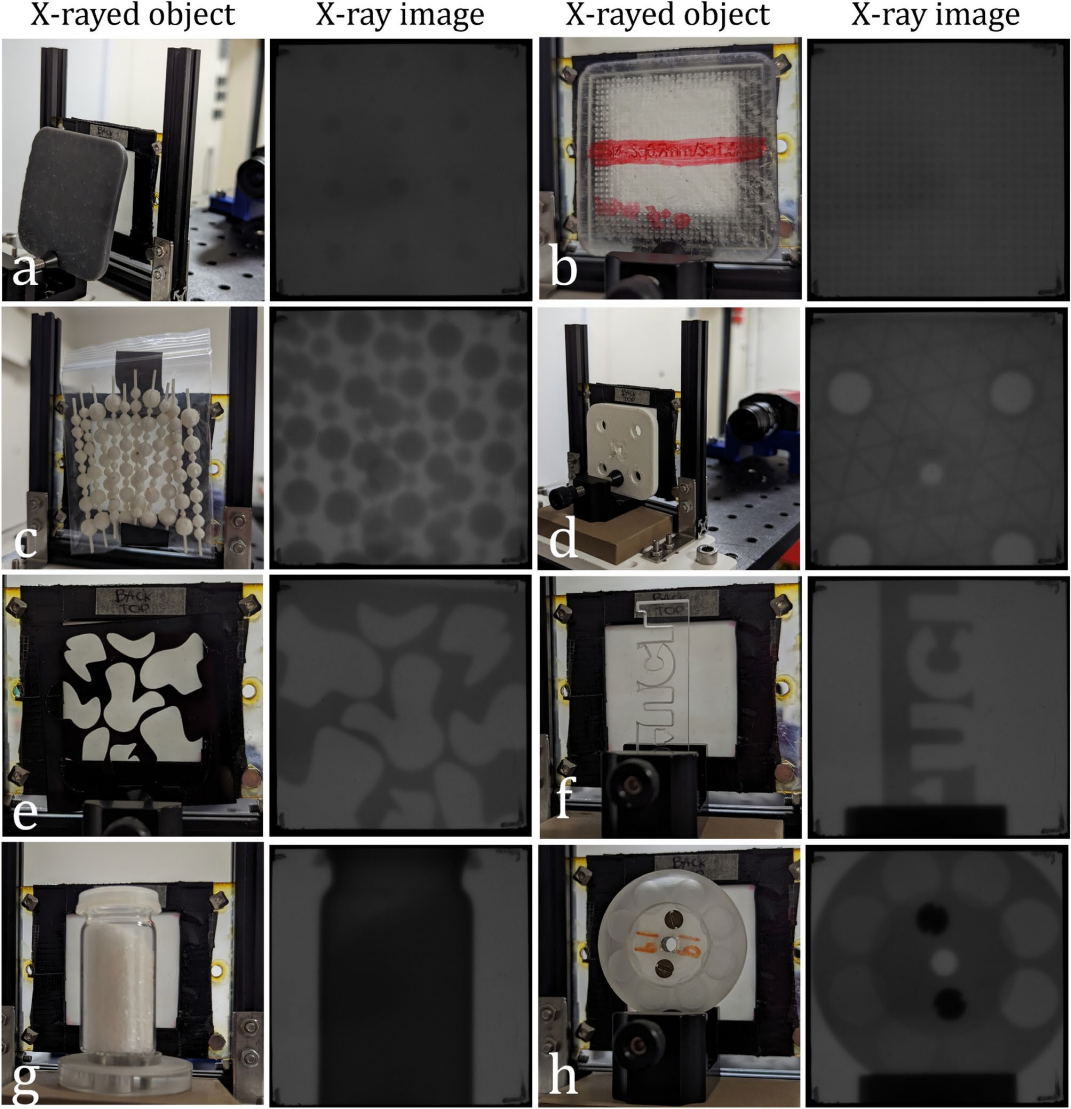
X-ray imaging. (a–h) Left image. X-rayed object (Tube voltage: 70 kVp, Exposure: 100ms). Right image. Uncorrected X-ray image. See Table 4 for more information about the material.
